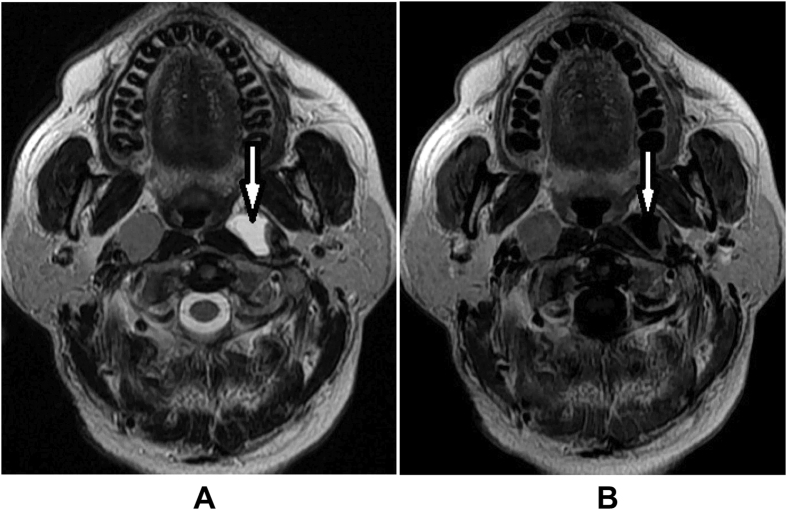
Appearance of a necrotic lymph node on MR images in a patient with NPC. (A) Axial T2-weighted (3300–5500/81–114) and (B) contrast-enhanced T1-weighted (400–600/9–16) MR images in a 45-year-old man show a left retropharyngeal lymph node with necrosis (arrows).
